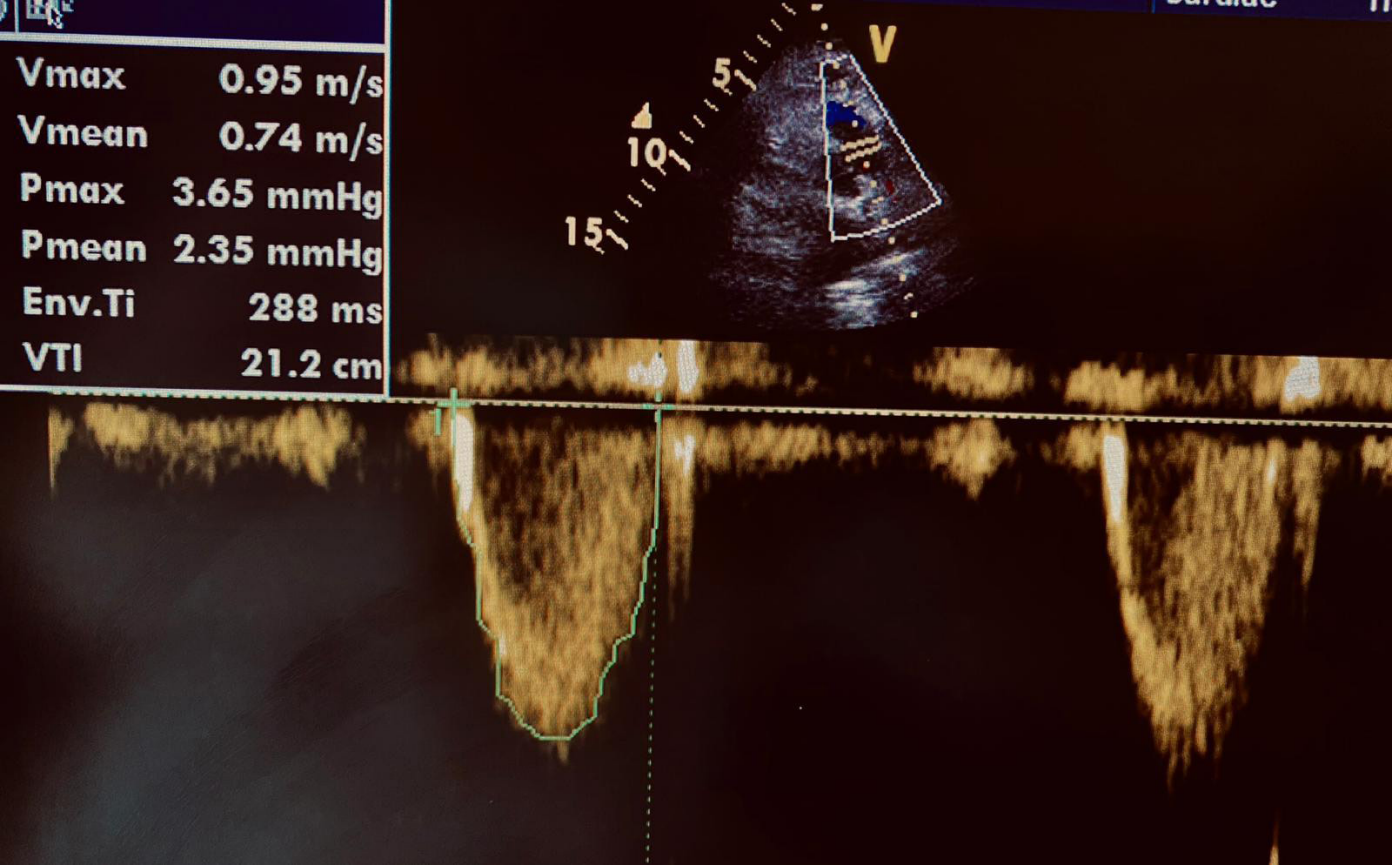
Pulse wave Doppler of left ventricular outflow tract (LVOT). The trace measuring LVOT velocity time integral (VTI)
